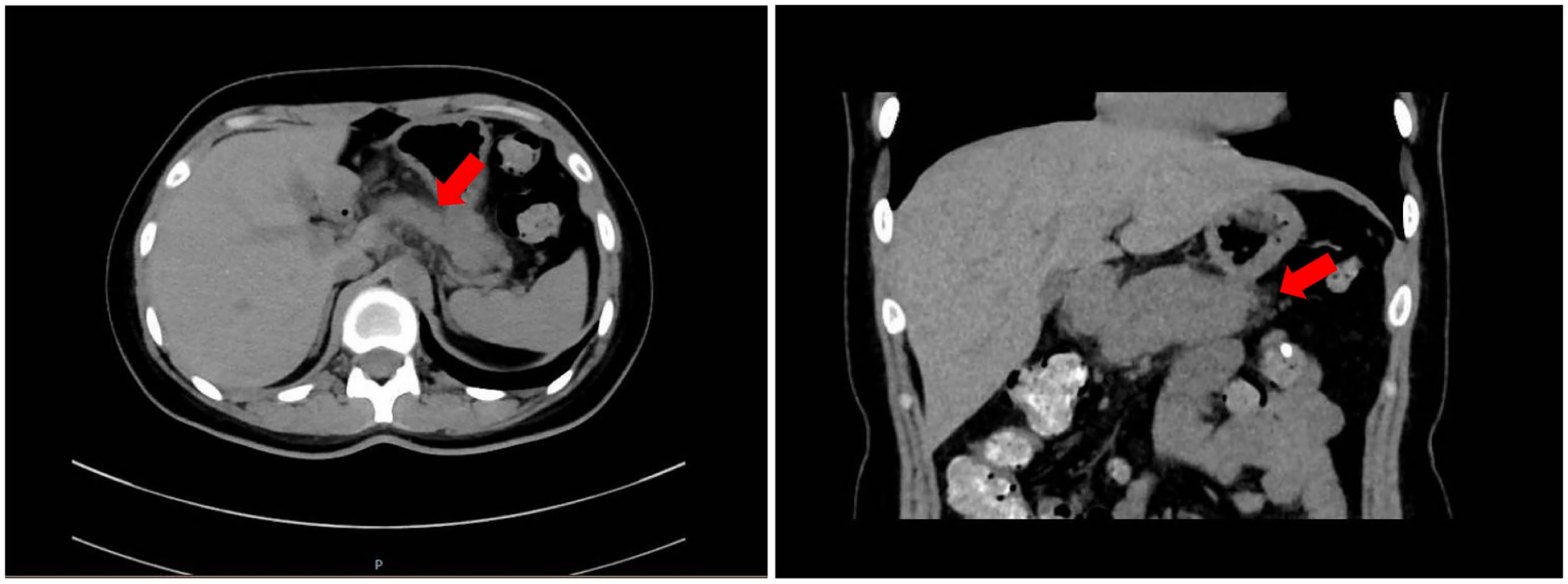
Abdominal CT (7 March 2024) showing ill-defined peripancreatic fat stranding (arrows) and mild enlargement of the pancreatic body (arrowhead); no biliary dilatation or cholelithiasis was present.
